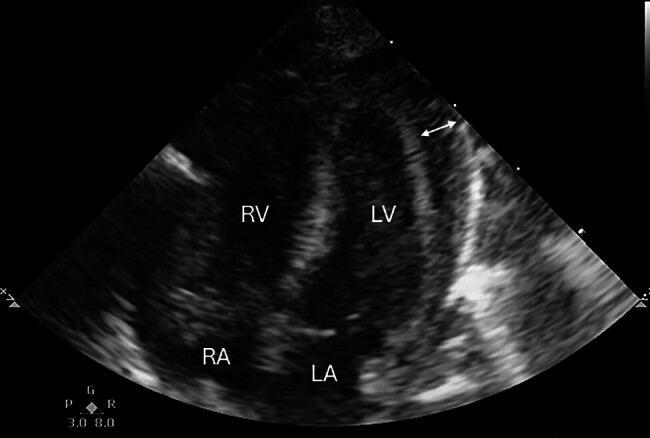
Apical 4-chamber view of echocardiogram showing the presence of pericardial effusion (pericardial effusion indicated by arrow). LA, left atrium; LV, left ventricle; RA, right atrium; RV, right ventricle.
